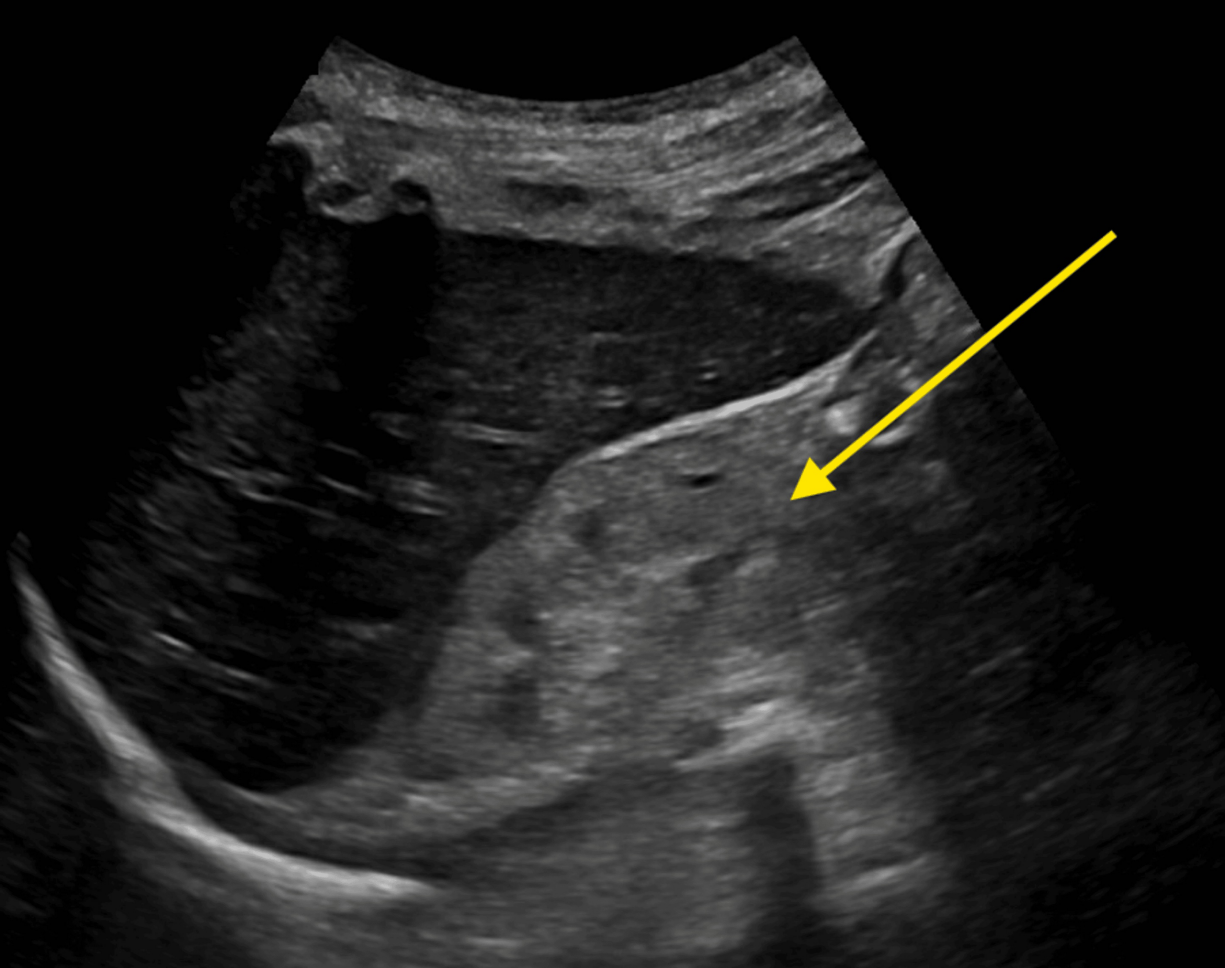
Retroperitoneal kidney ultrasound The retroperitoneal kidney ultrasound showed no signs of hydronephrosis.
